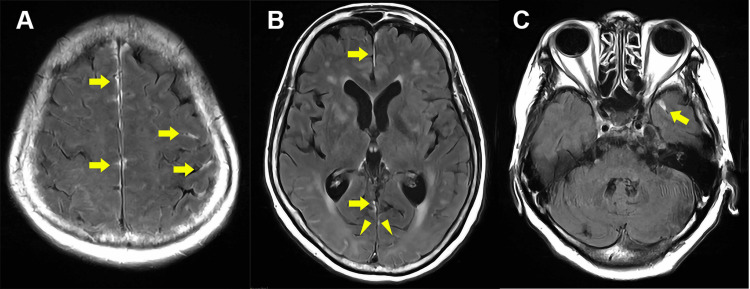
Contrast-enhanced head MRI Contrast-enhanced fluid-attenuated inversion recovery (FLAIR) axial images (A-C) demonstrate abnormal enhancement along the gyri of the interhemispheric frontal and parietal lobes, as well as within the sulci of the frontal and temporal lobes (arrows) and high T2 signal intensity without contrast enhancement in the cerebral white matter, predominantly in the occipital lobe (arrowheads) MRI: magnetic resonance imaging
